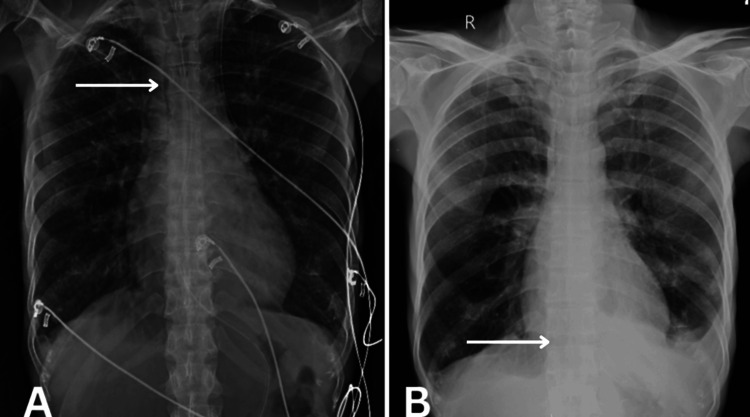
X-ray showing (A) increased mediastinal widening and (B) mild obliteration of angle
